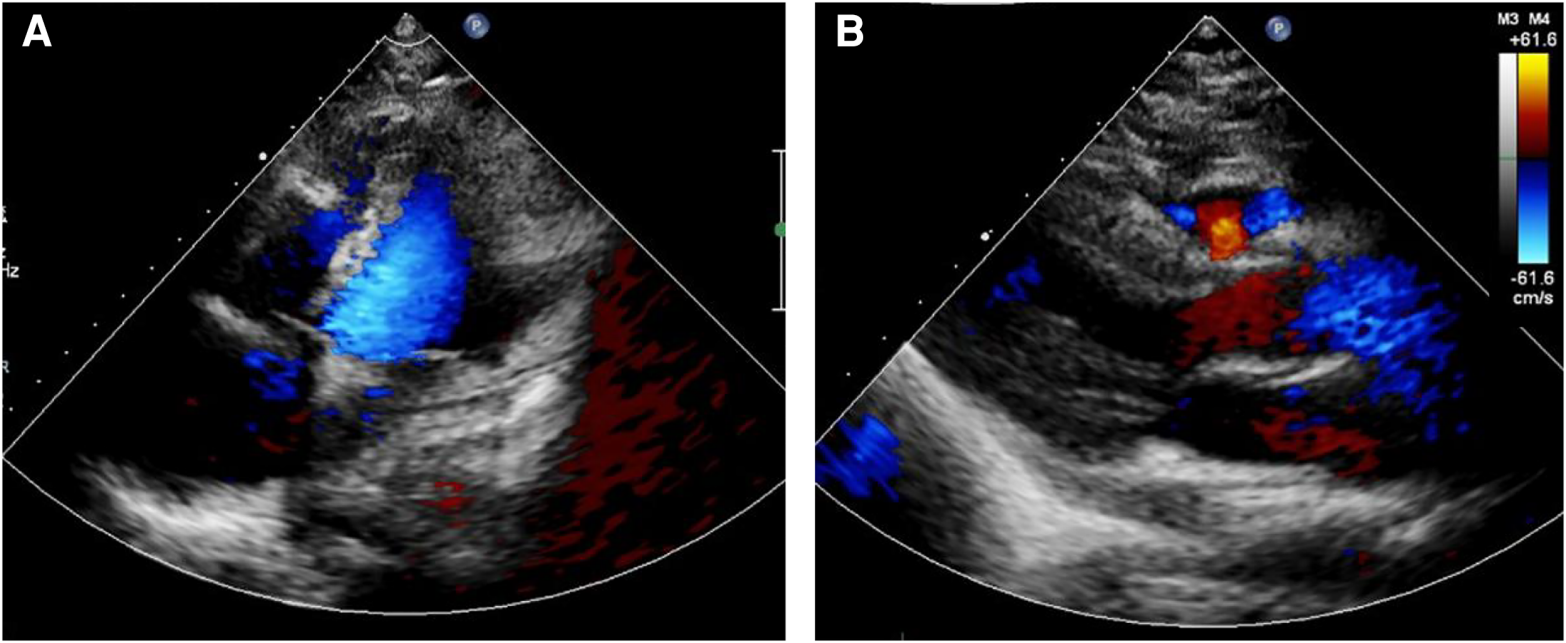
Postoperative echocardiography showed that the right atrial mass was totally resected (A), no hemodynamic abnormality (B) and no recurrence signals.
